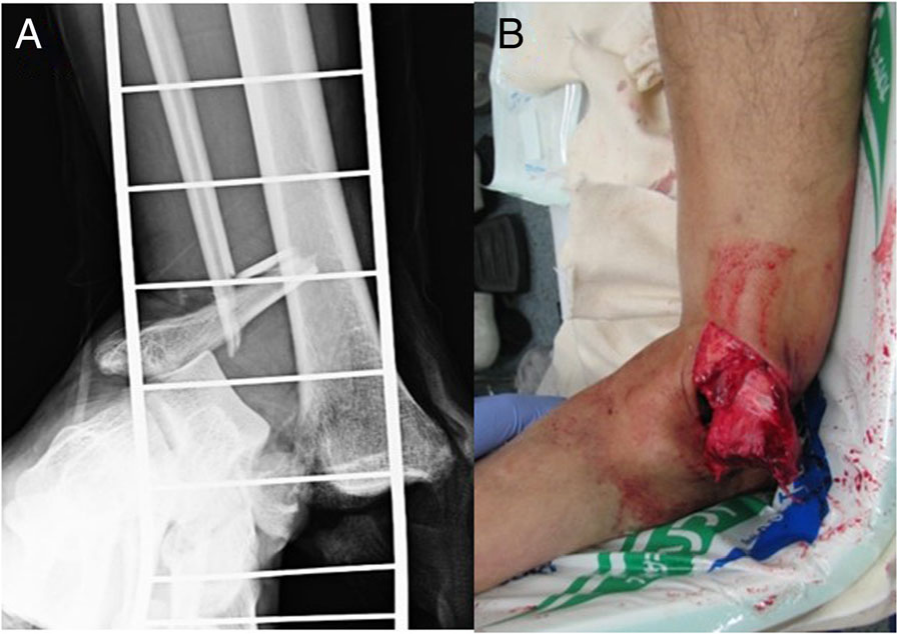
Anteroposterior radiography (a) and physical appearance (b) of the *right ankle*. It was classified as a Gustilo–Anderson 3A, AO classification 44-C2 open fracture
